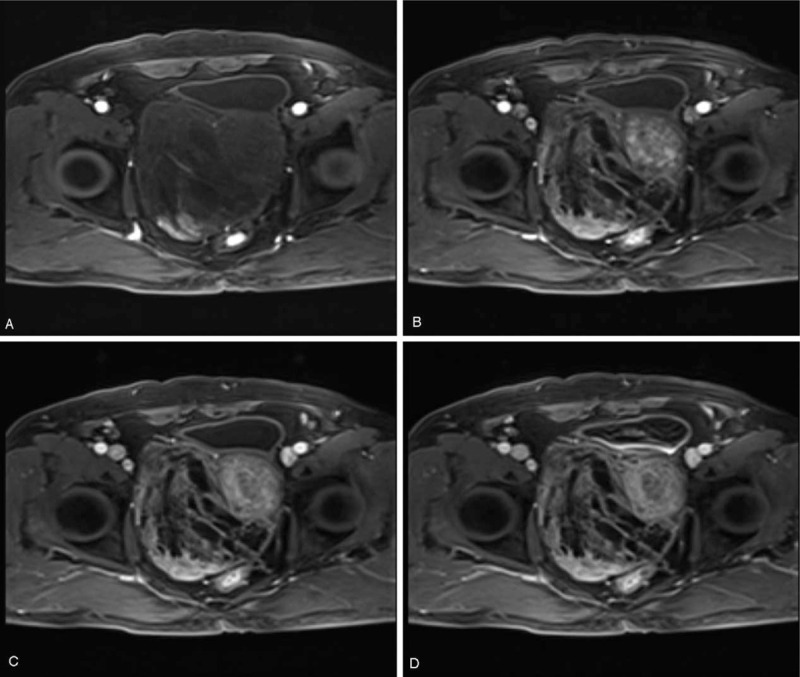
DCE-MRI manifestation of GMPC. On DCE-MRI, the solid part of the tumor showed obvious enhancement, and the cysts showed nonenhancement during arterial (A) phase to delayed phase (D). MRI = magnetic resonance imaging, DCE-MRI = dynamic contrast-enhanced MRI, GMPC = giant multilocular prostatic cystadenoma.
